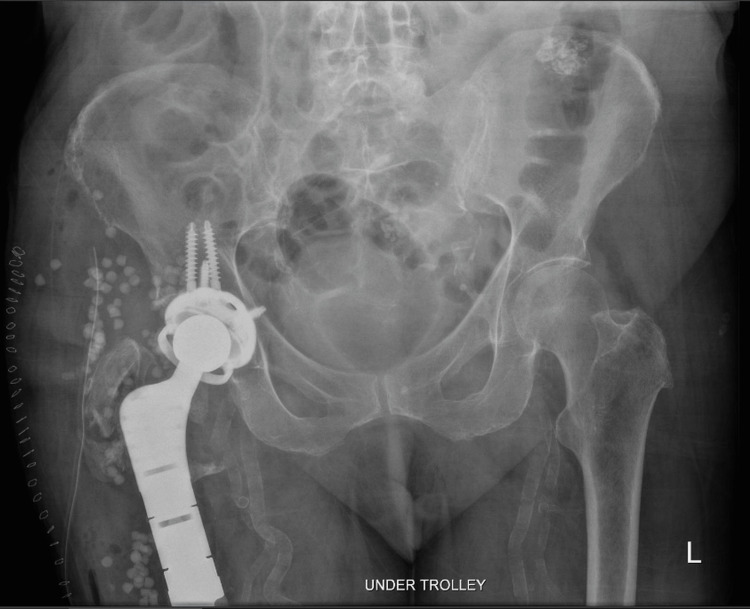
Immediate post-operative anteroposterior pelvis radiograph following right-sided cemented DePuy Synthes modular proximal femoral replacement An anteroposterior pelvis radiograph taken on the same day of surgery demonstrating a right-sided cemented DePuy Synthes modular proximal femoral replacement with an uncemented DePuy Synthes Pinnacle acetabular cup and constrained liner. The femoral stem is well-seated within the cement mantle, and multiple screws secure the acetabular reconstruction cage. The greater trochanter was preserved and reattached through the prosthesis to restore abductor integrity. Prophylactic cerclage wiring was applied around the bone-implant interface for additional construct stability. Antibiotic-loaded STIMULAN beads are visible around the implant site. The right hip joint remains native and intact.
